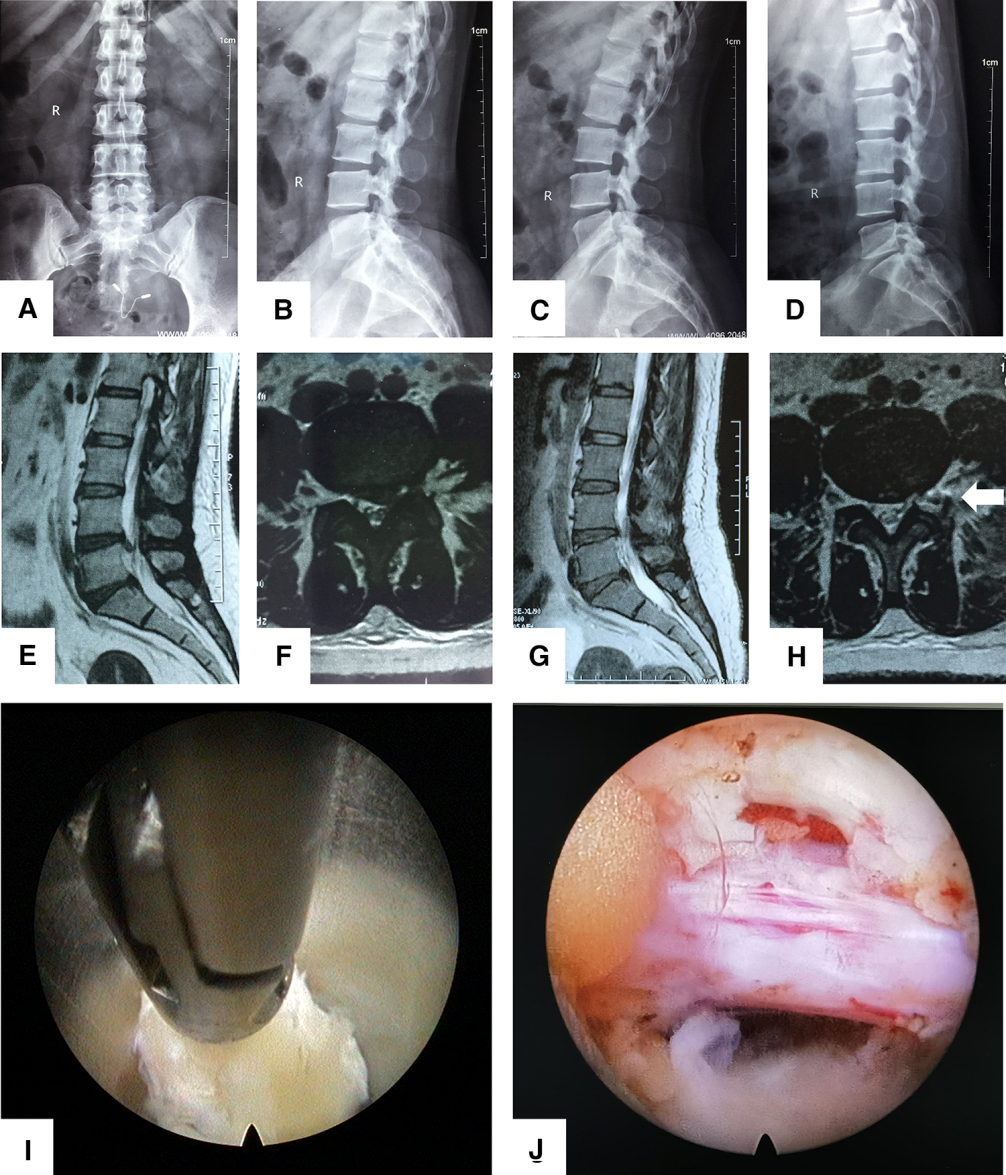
A 34-year-old female patient had radiating pain in the left lower extremity. (A-D): She underwent lumbar anteroposterior and lateral x-ray radiographs and lumbar overflexion-extension x-ray radiographs. The imaging data showed no lumbar instability. (E-F): Preoperative axial and sagittal MRI (T2WI) results showed L4/5 LDH with nerve root compression. (G-H): The postoperative MRI scans (1 day after surgery) of this patient show that the herniated intervertebral disc resection was satisfactory, and the structure of the lumbar facet joint was fully preserved (white arrow). (I-J): The herniated intervertebral disc was removed under endoscopy.
